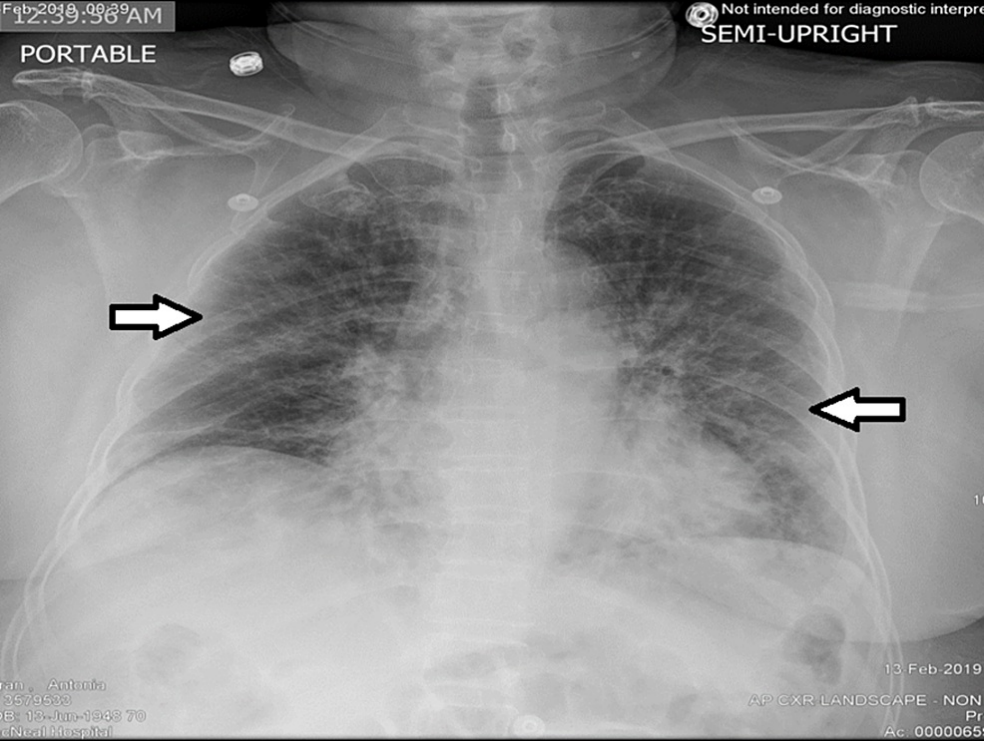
Chest X-ray on hospital day 3 showing diffuse bilateral infiltrates.
